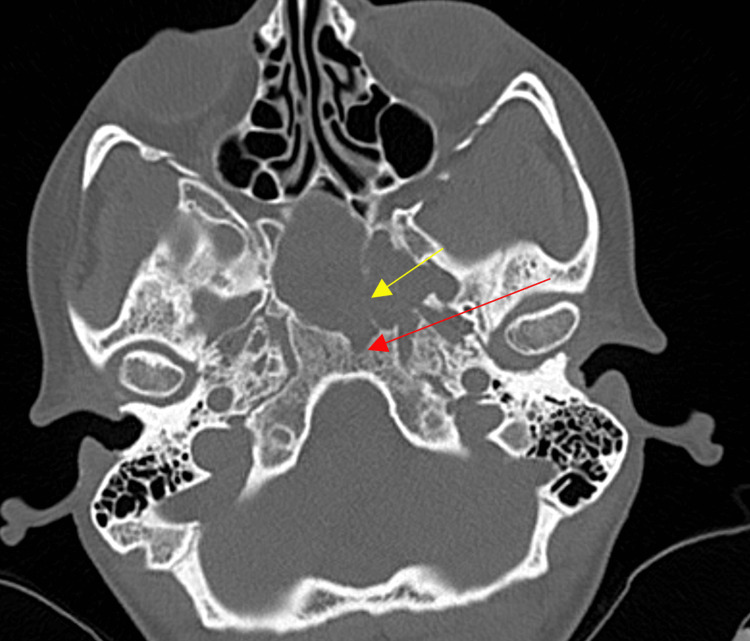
Axial non-contrast computed tomography of the head bone window. Bone window showing compression and partial erosion of the left sphenoid bone (yellow arrow) and the clivus (red arrow).
